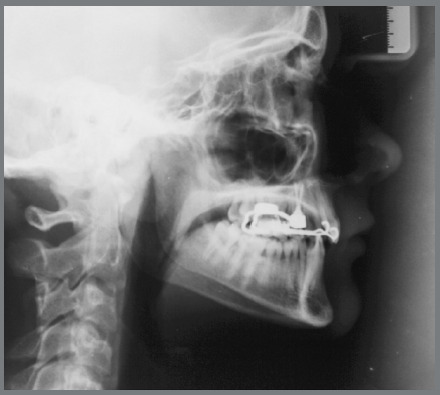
Lateral radiograph after first orthopedic phase.
